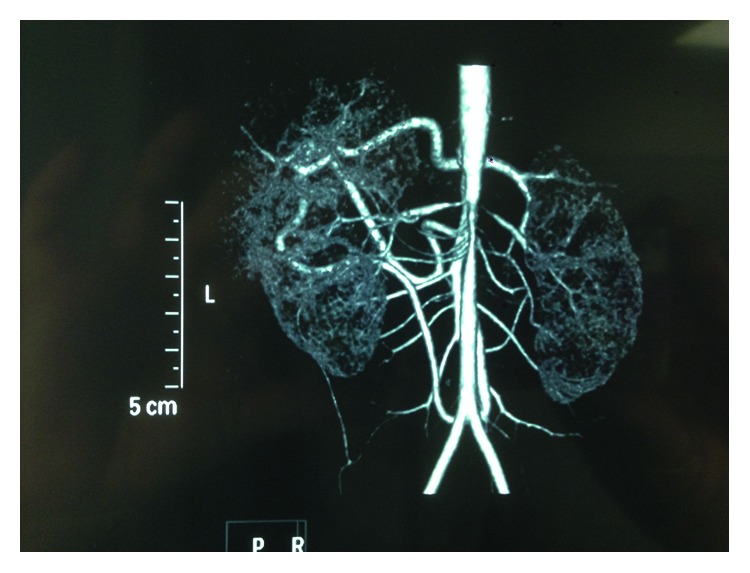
CTA at diagnosis showed severe segmental aortic stenosis arising before the origin of the upper mesenteric artery extending for a length of 3–4 cm with lumen diameter of 0.2–0.3 cm at the narrowest part and a poststenotic diameter of 0.6–0.7 cm. Two right renal arteries were demonstrated, both with ostial stenosis, as well as an auxiliary stenotic artery perfusing the lower pole of the right kidney. Left renal artery also presented ostial stenosis. Furthermore, an enlarged riolan arcade resulted in an increased blood flow to the upper mesenteric artery from the lower mesenteric artery providing collateral circulation.
